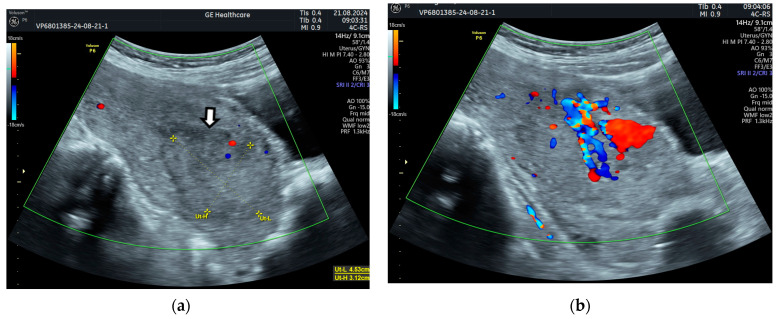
Vaginal ultrasound image of the uterus: (a) arrows show the lower part of the uterine cavity filled with a homogeneous content (hematometry), with a hypoechoic linear zone at the upper edge adjacent to the myometrium; (b) vascular formations in the uterine cavity on color Doppler.
